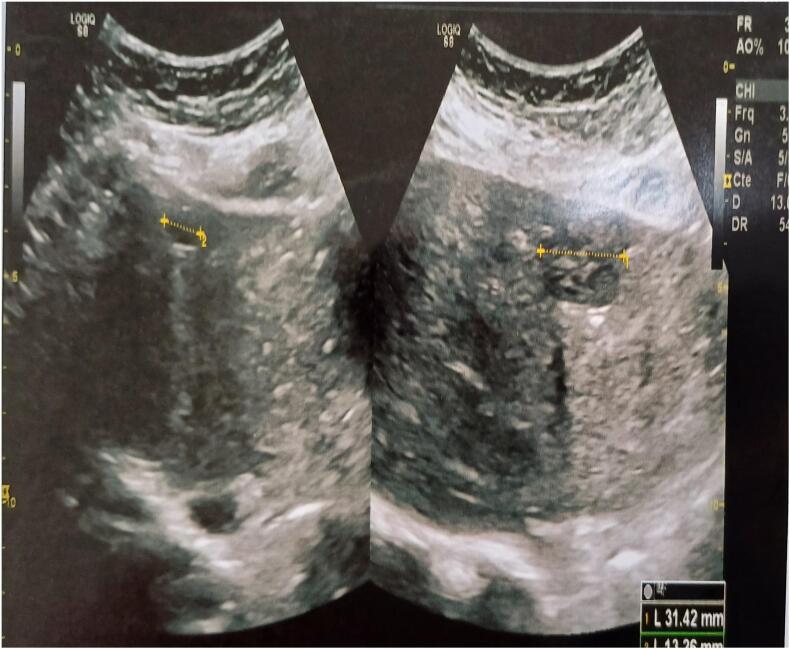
Abdominal ultrasound demonstrates two hypoechoic, heterogeneous nodular lesions with regular margins in a liver of normal volume. The larger lesion (31 mm) is located in segment IV, while the smaller (20 mm) is in segment VIII.
